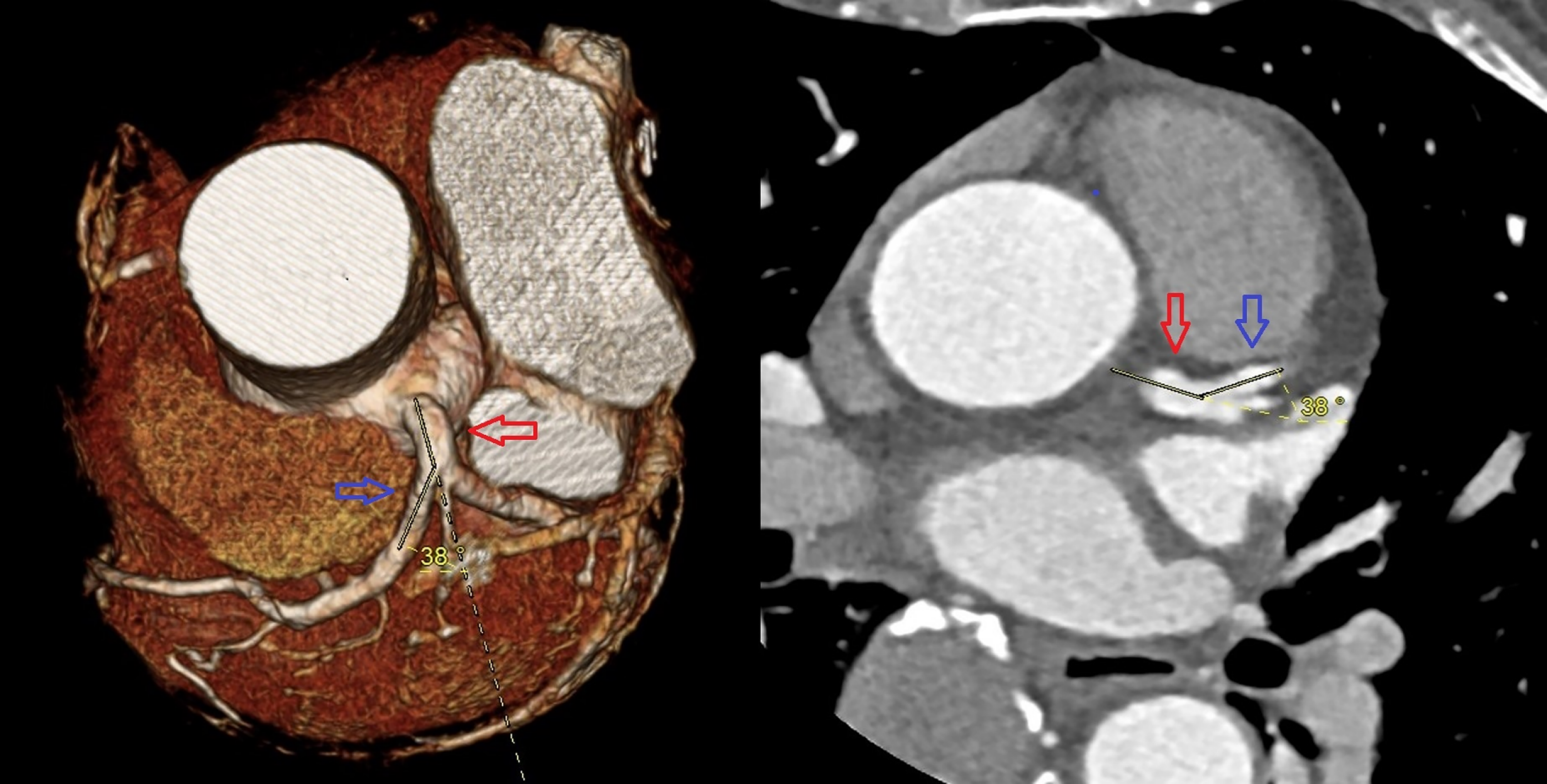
A representative image of angle measurement on computed tomography angiography. The angle between the left main coronary artery (red arrow) and the left anterior descending coronary artery (blue arrow) is 38°.
